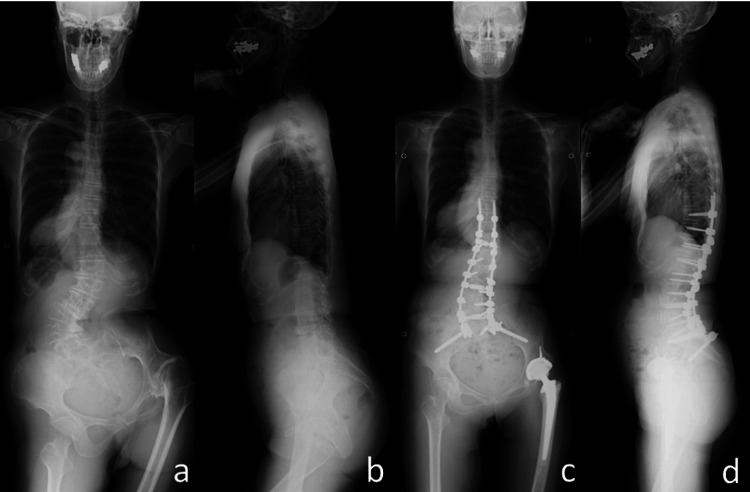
Preoperative and postoperative whole-spine posteroanterior radiographs. Patient standing supported by the entire soles of bilateral feet. Preoperative whole-spine posteroanterior radiographs showing rigid lumbosacral and thoracolumbar scoliosis and severe PO accompanied by ankylosis of the TB-affected hip on the right side (a). Preoperative lateral radiograph showing loss of lumbar lordosis and hip flexion contracture (b). The final radiograph shows almost normal alignment on the sagittal and coronal planes, significant improvement of PO, and adduction hip contracture (c and d).
